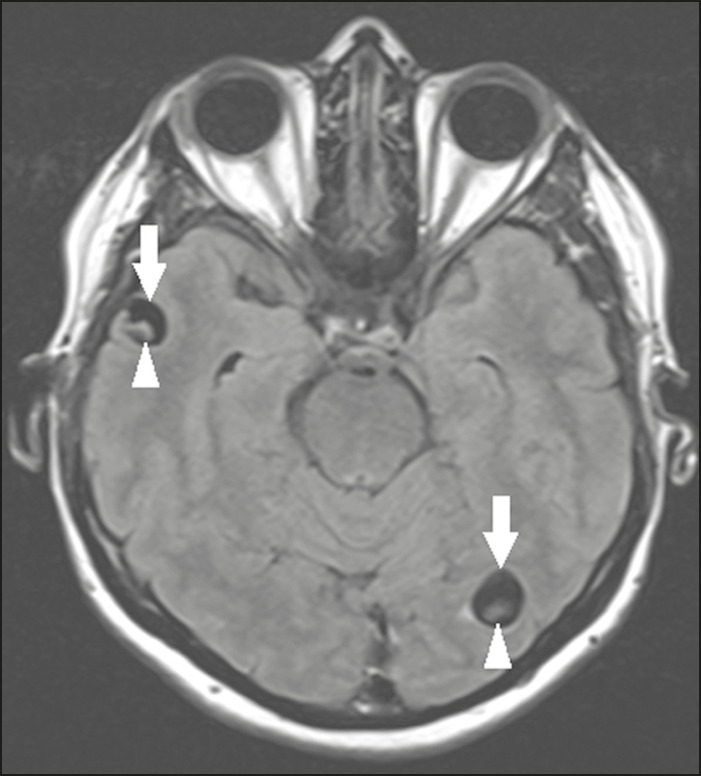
Neurocysticercosis. Axial FLAIR MRI showing cyst-like formations with signal intensities similar to that of the CSF, affecting the right temporal and left occipital lobes (arrows), and containing scolices (arrowheads). There is no evidence of perilesional edema, the absence of which is characteristic of the vesicular stage.
